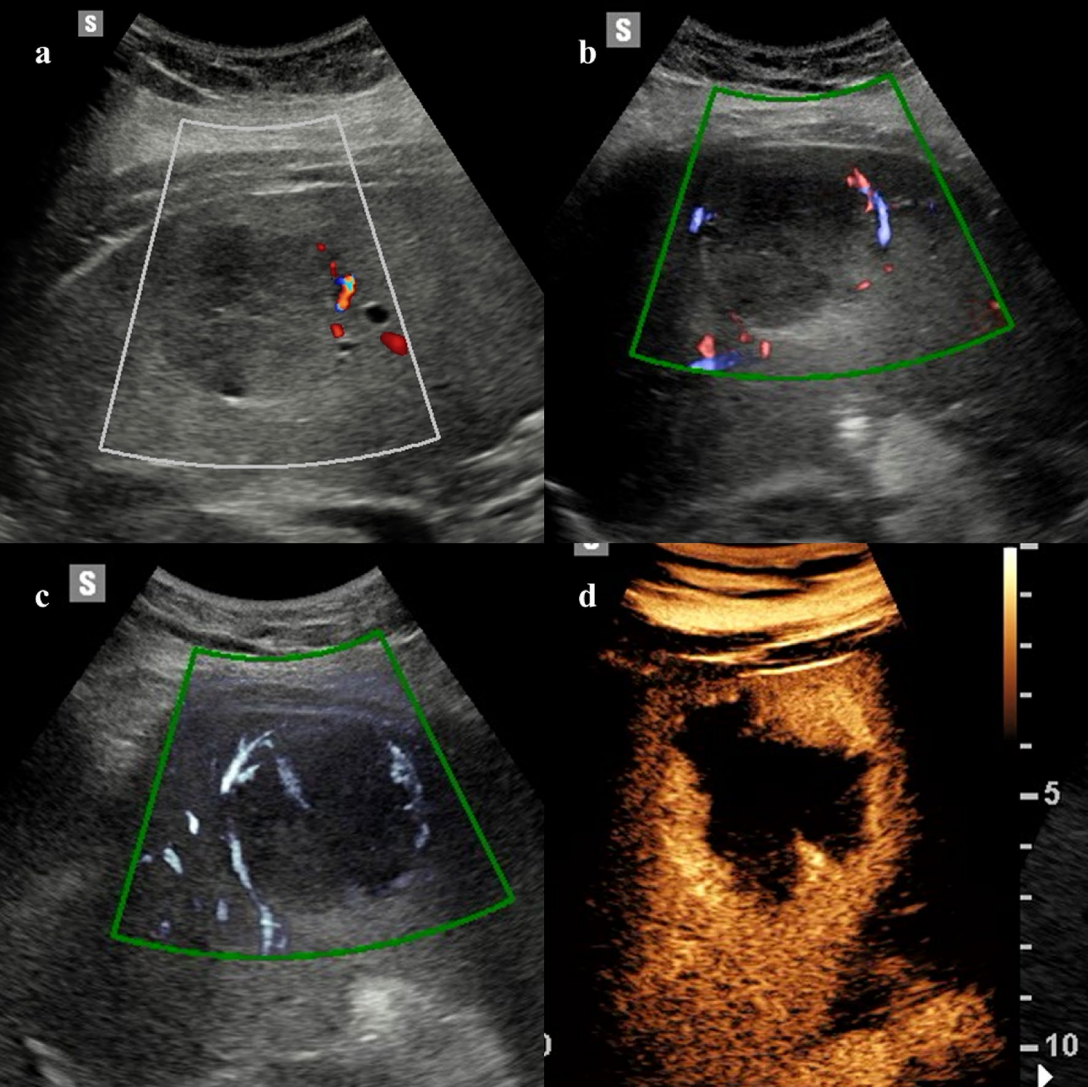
40-year-old woman with a 5.7 cm hepatic hemangioma incidentally detected on abdominal ultrasound examination. Color (a) and directional power Doppler (b) imaging demonstrate minimal peripheral vascularization. Microvascular flow imaging shows a strip rim pattern of vascularization (c). Contrast-enhanced ultrasound (CEUS) confirmed the diagnosis of hepatic hemangioma by showing the peripheral globular enhancement (d, 63 s after contrast injection)
